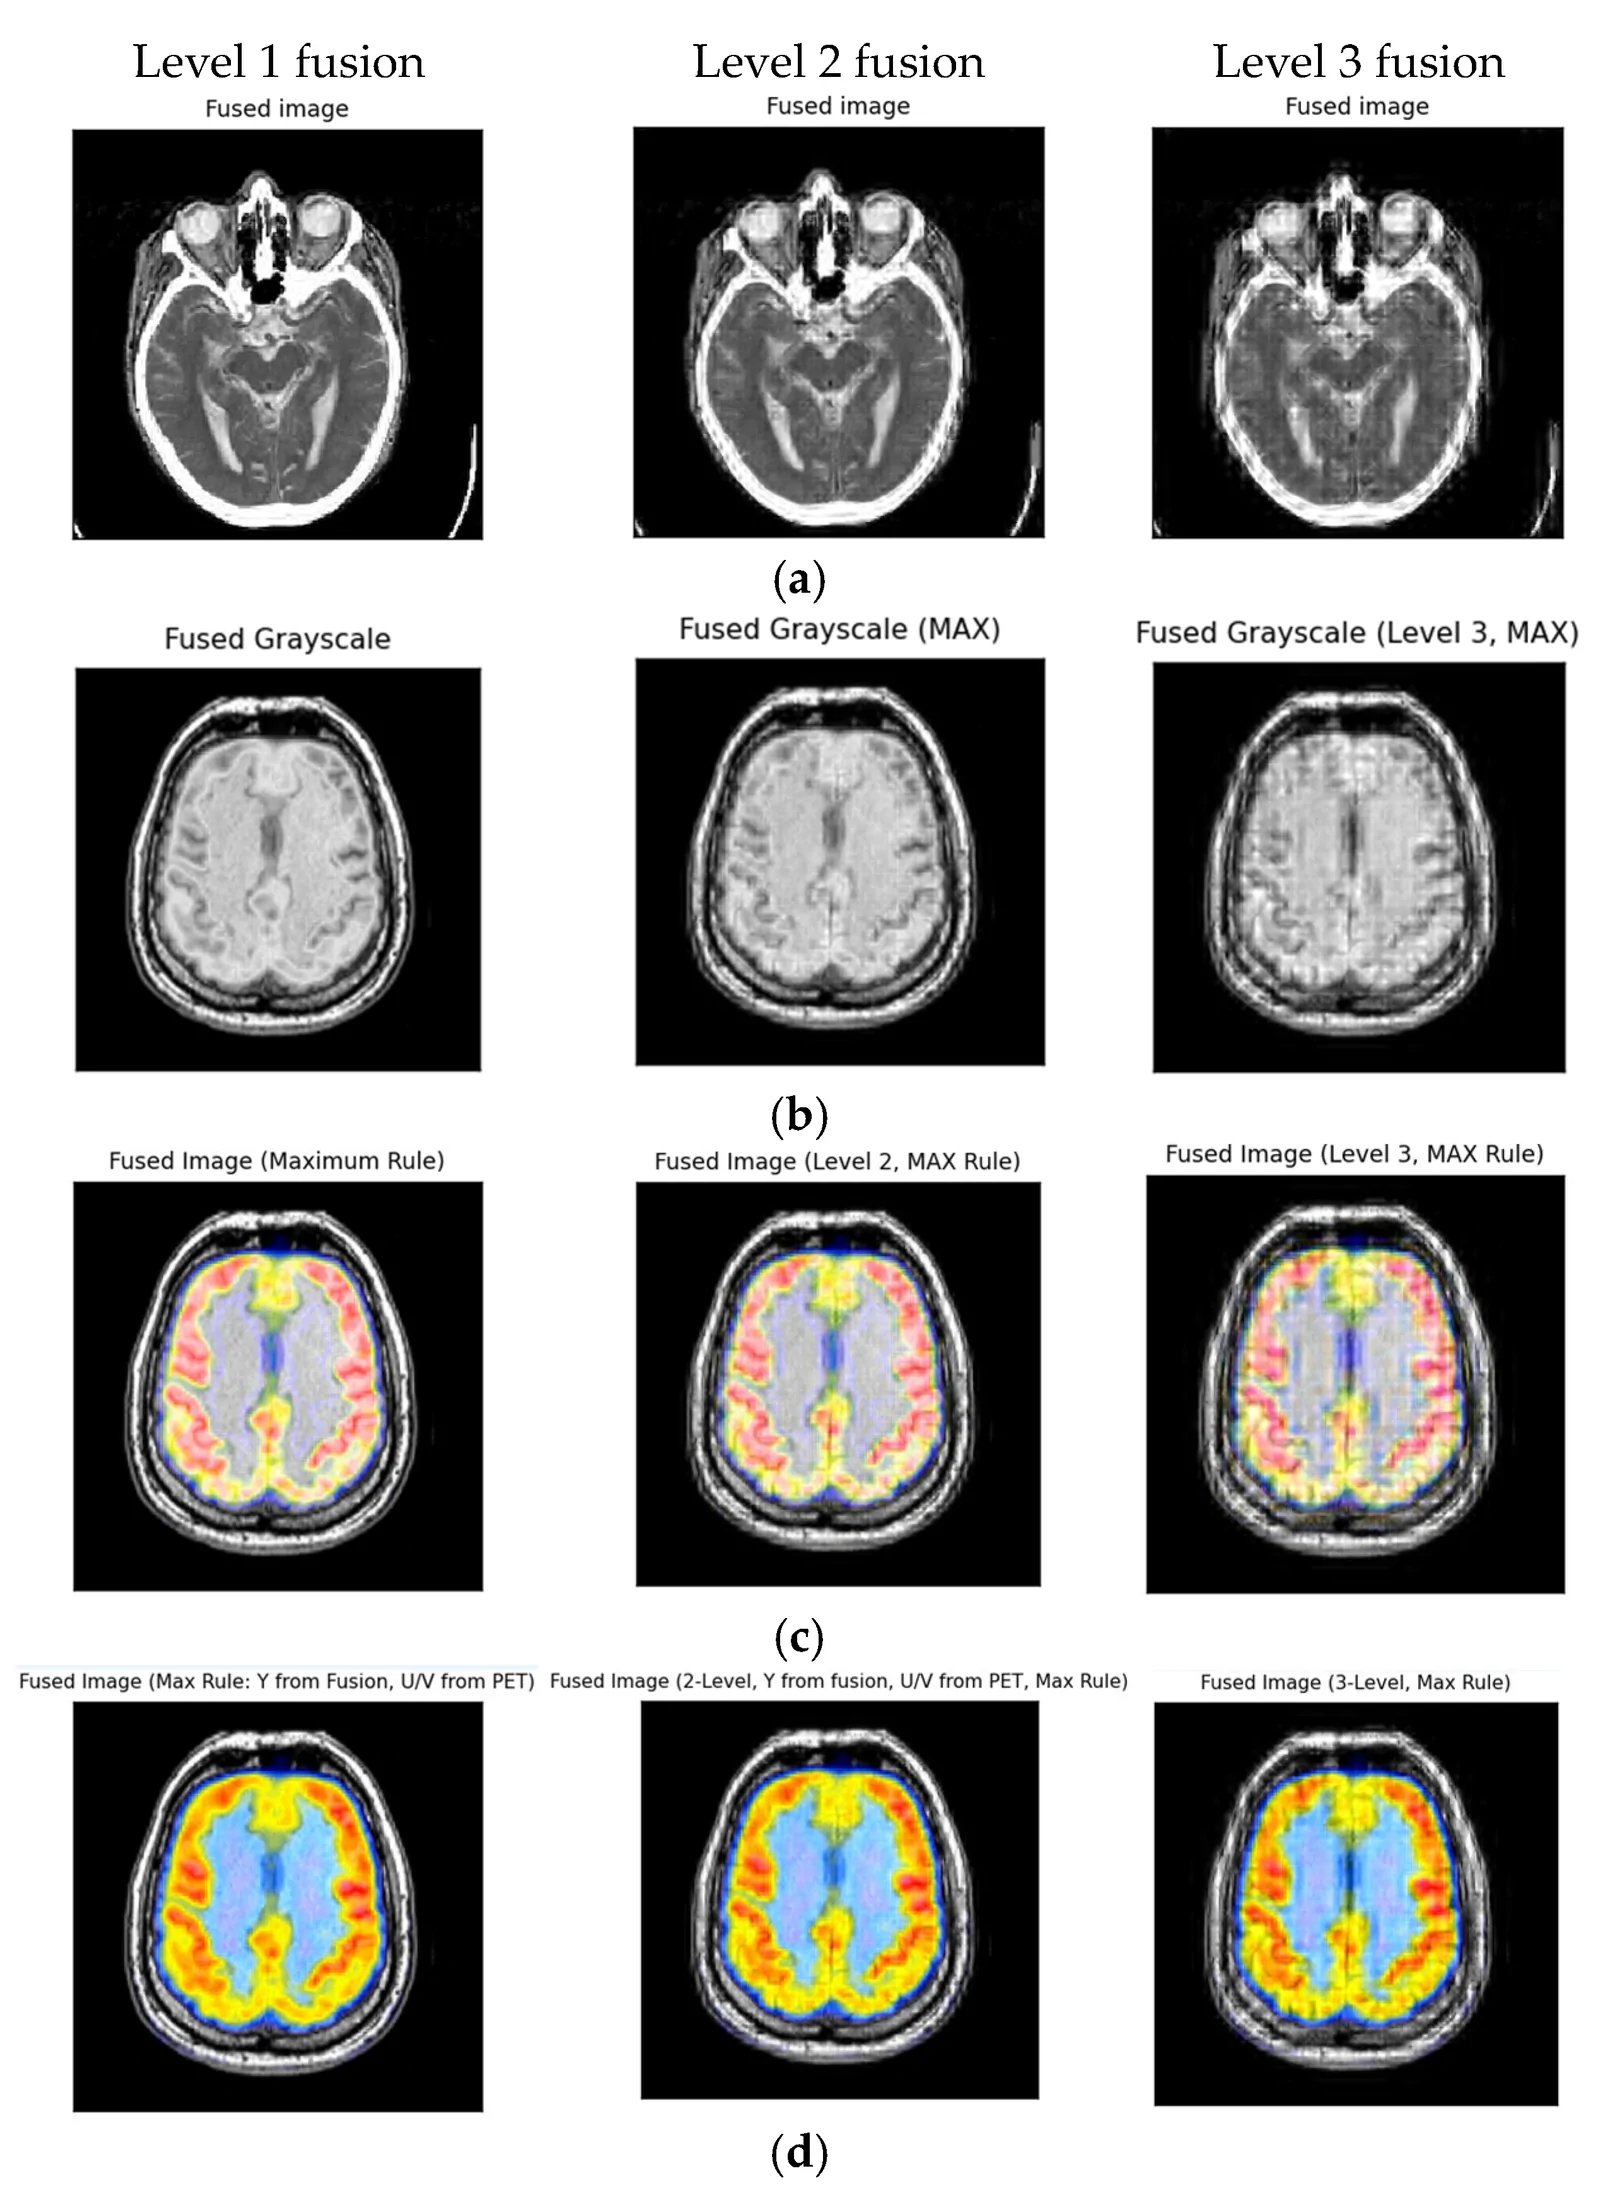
Comparison of one-, two-, and three-level fusion results, using the maximum fusion rule with the ‘bior4.4’ wavelet: (a) CT and MRI; (b) PET grayscale and MRI; (c) PET without conversion and MRI; (d) PET YUV conversion and MRI.
